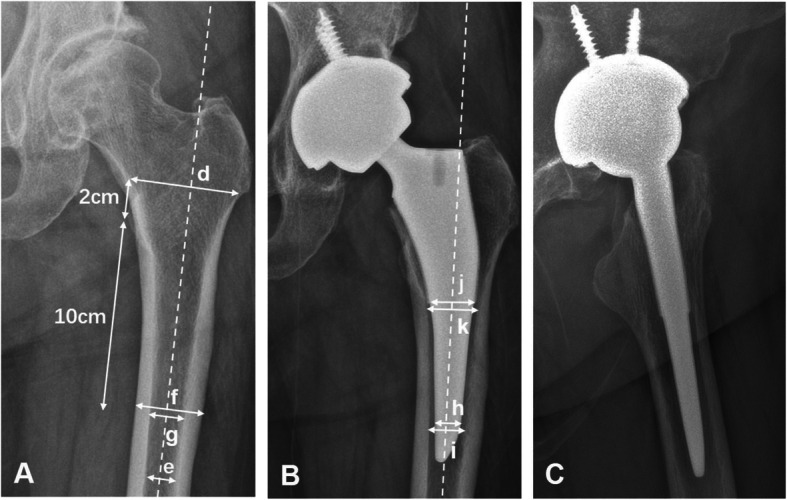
a–c A representative image for type II fit. This patient was a 66-year-old woman who underwent THA at age 65 using a short tapered stem. a A preoperative AP radiograph of her left hip. b A 6-month postoperative AP radiograph of the hip. c A 6-month postoperative lateral radiograph of the hip. The following parameters were measured: (*d*) metaphyseal diameter 2 cm above the level of the lesser trochanter midpoint, (*e*) isthmus diameter which represents the width of the narrowest part of the proximal femoral canal, (*f*) diameter of the femoral shaft which is measured 10 cm distal to the center of the small trochanter, (*g*) internal width of the medullar canal which is measured 10 cm distal to the center of the small trochanter, (*h*) width of the stem which is measured at the proximal end of stem tip arc, (*i*) internal width of the medullar canal which is measured at the proximal end of the tip arc, (*j*) width of the stem which is measured at the distal end of porous coating, and (*k*) internal width of the medullar canal which is measured at the distal end of porous coating
